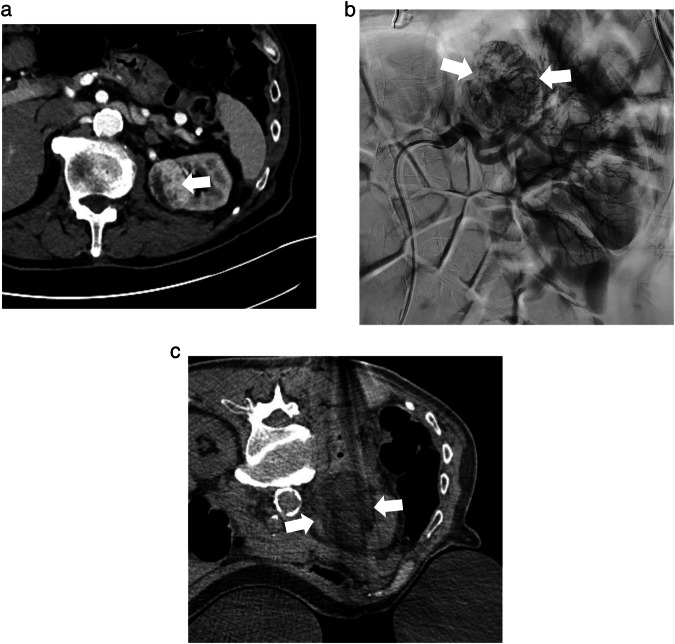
Eighty-three-year-old man with a 4.2 cm biopsy-proven clear cell RCC in the left kidney on contrast-enhanced CT (a, white arrow). DSA of the left renal artery shows the hyper-vascular mass in the upper pole of the left kidney (b, white arrows). Axial CT obtained during percutaneous CA the following day shows the hypodense iceball surrounding the mass in the left kidney (c, white arrow)
